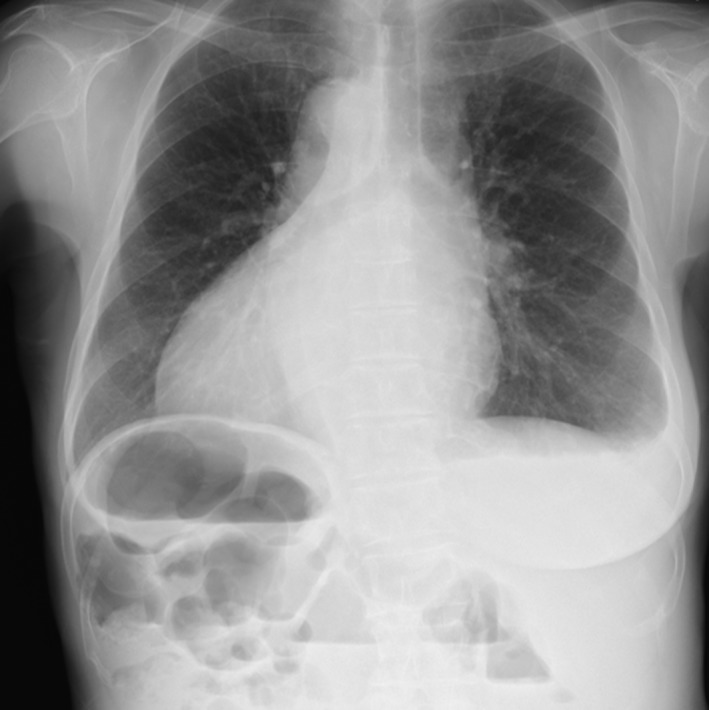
Chest radiography showing dextrocardia.
